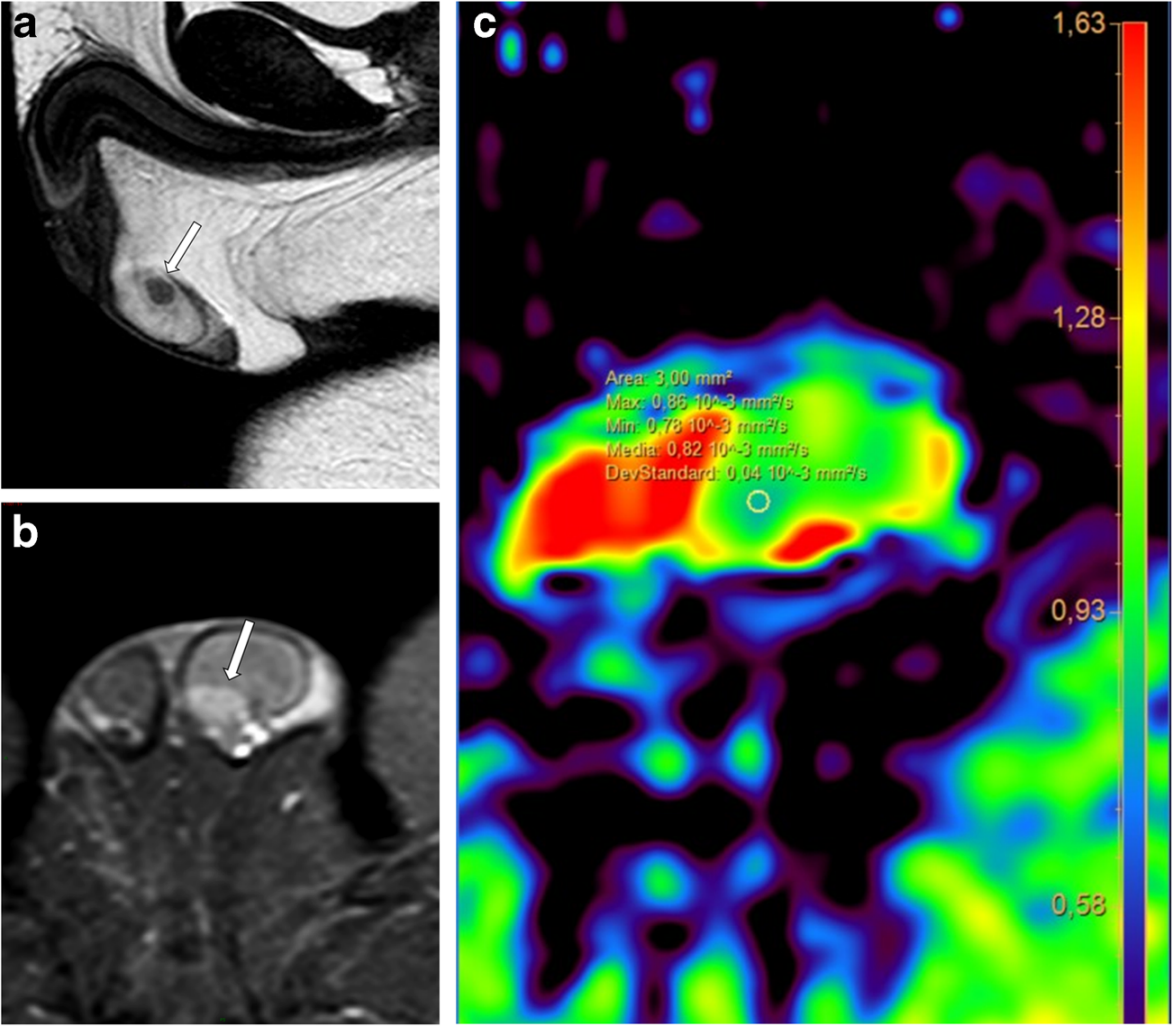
a-c MR study in a case of benign Leydig cells tumour. a T2-weighted TSE sequence, sagittal view and b post-contrast T1-weighted SPIR TSE sequence, axial view, depicting a round hypointense on T2-wi testicular focal lesion (arrow), with homogeneous hyperenhancement. c ADC map
